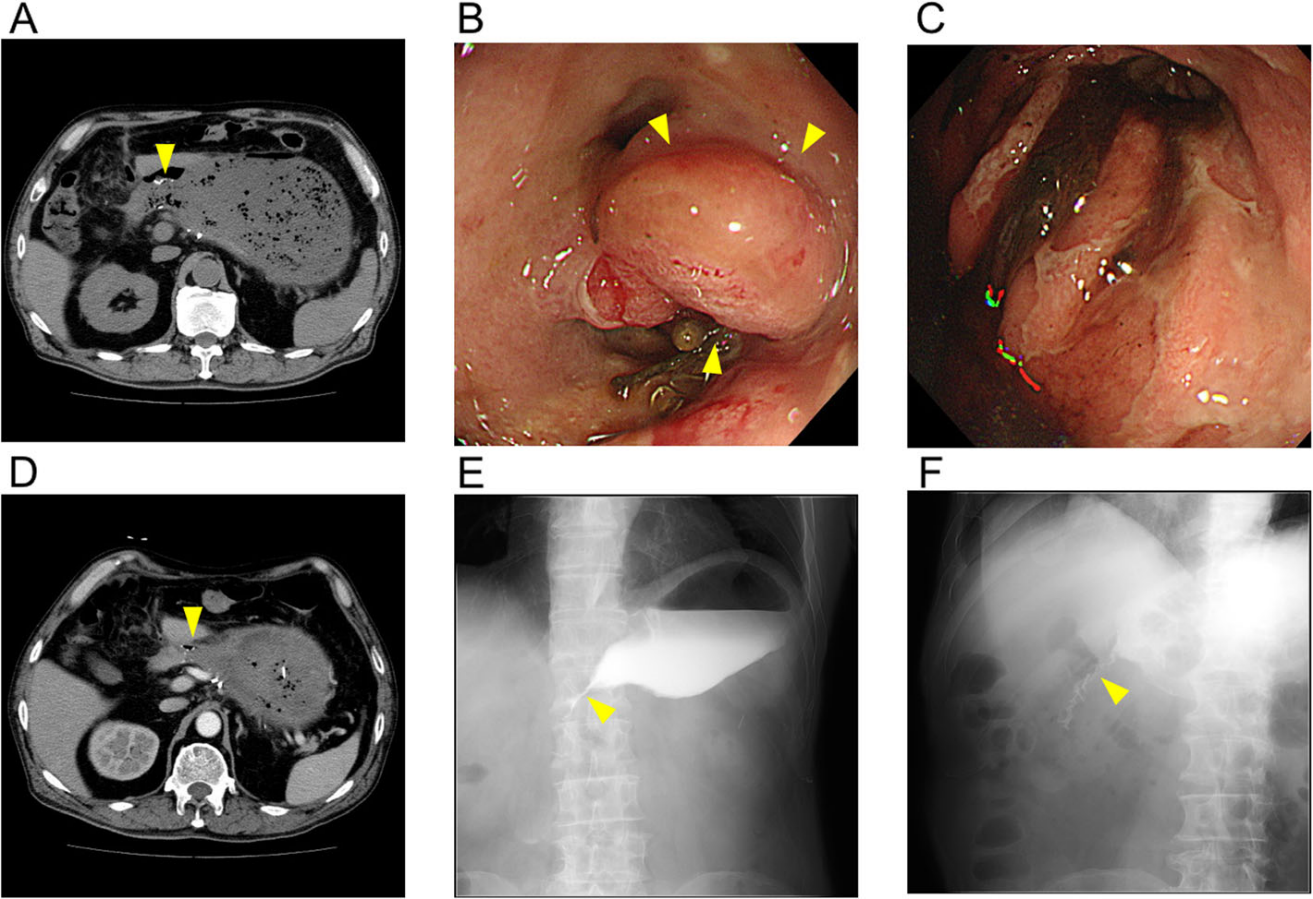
Representative images before systematic steroid treatment. a Computed tomography (CT) scans on postoperative day 9. The yellow arrowhead indicates the anastomotic part. b Endoscopy showing extreme swelling, like a polyp, in the duodenum on postoperative day 19. Yellow arrowheads indicate swelling. c Endoscopy showing the remaining portion of the stomach was edematous on postoperative day 19. d CT scan shows the wall of the anastomotic part was edematous, and swelling persisted on postoperative day 20. The yellow arrowhead indicates the anastomotic part. e Fluoroscopy revealing little contrast medium could flow into the duodenum on postoperative day 26. The yellow arrowhead indicates the contrast medium. f The quantity of contrast medium that could flow into the duodenum did not change after local injection of steroid on postoperative day 56. The yellow arrowhead indicates the contrast medium
